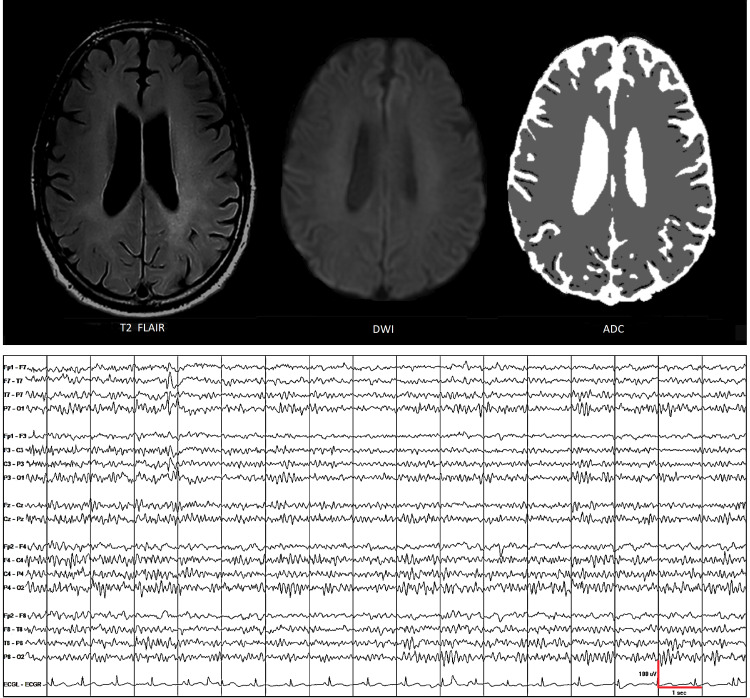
During his clinic follow-up, approximately two and a half months after the overdose, brain MRI showed near-complete resolution of white matter hyperintensity and EEG showed irregular theta and delta waves anteriorly and normal alpha rhythm posteriorly. Epileptiform sharp waves occurred infrequently over the left temporal head region, but this finding is not new (the patient has mesial temporal lobe epilepsy).
